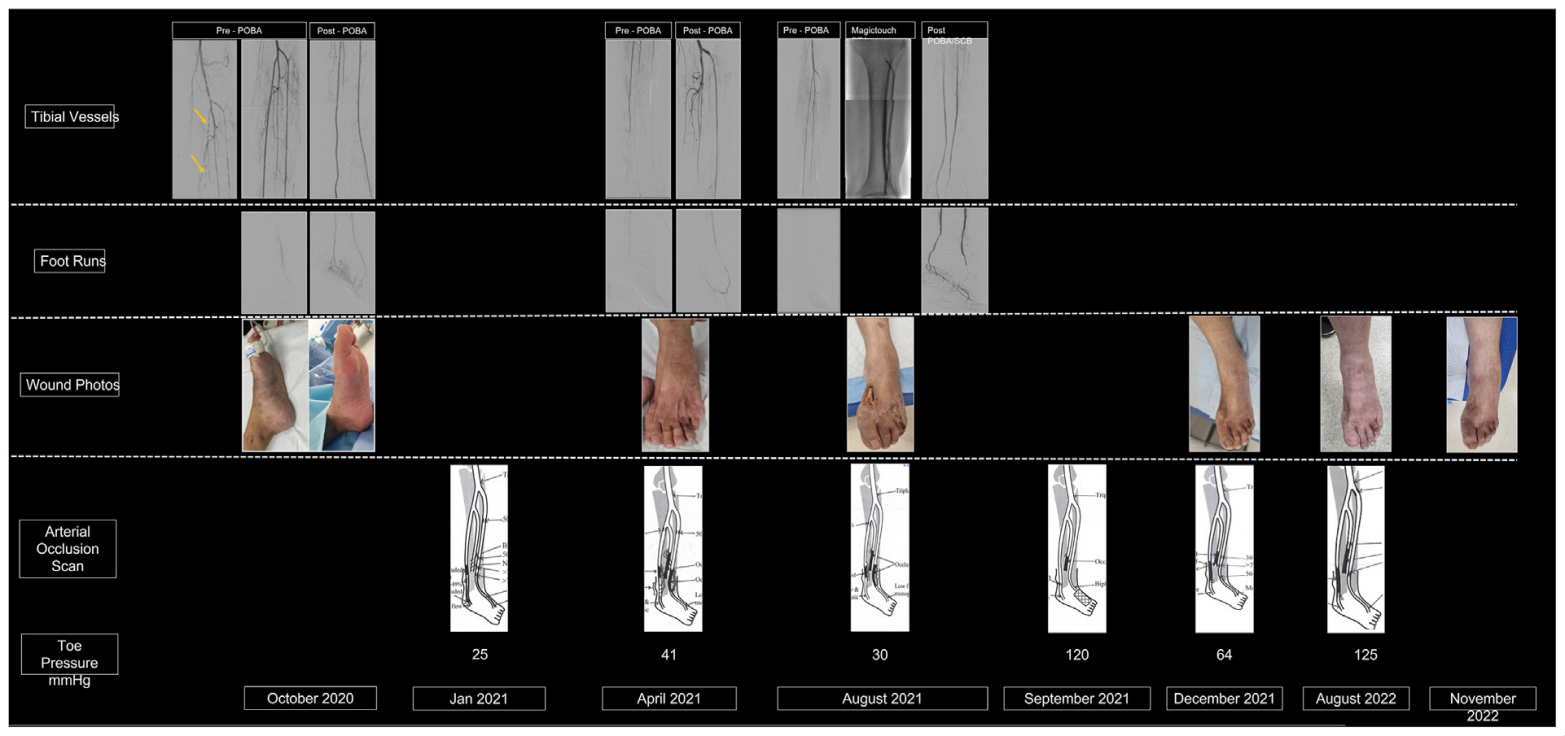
Timeline of patient with polyarteritis nodosa (PAN)-induced critical limb threatening ischemia (CLTI). She first presented with left acute limb ischemia with a mottled dusky foot and required emergent angioplasty to restore perfusion. Intraoperatively, angiographic evidence of “beading” with alternating dilatation and narrowing was observed (*yellow arrow*). Sequential angiography also revealed disease progression, with worsening distal tibial vessel patency and the last angiography demonstrating a “desert foot.” During her disease, she continued to deteriorate and experienced CLTI with tissue loss, requiring repeated angioplasty over a span of 10 months. In her third angioplasty, we used the MagicTouch, a sirolimus-coated percutaneous transluminal angioplasty catheter, on the anterior tibial artery (ATA), in the hope of arresting the vasculitis progression with the anti-inflammatory and immunosuppressive properties of sirolimus. Her foot healed within 4 months after sirolimus treatment, and she remained intervention free at 17 months and ulcer free at 13 months after her last treatment. Surveillance arterial occlusion ultrasound scans at 1, 6 and 12 months demonstrate that her ATA remained patent with no occlusion. She continued to have a strong dorsalis pedis pulse at her last follow-up at 17 months.
